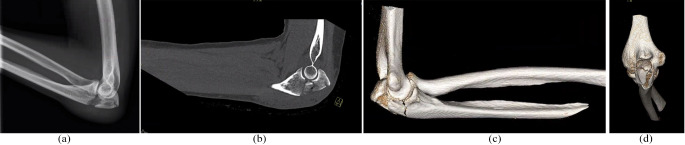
Preoperative imaging of a 47-year-old female with a comminuted and displaced Mayo II-B olecranon fracture (case 24, Table 1). (a, b) Lateral radiograph and sagittal computed tomography (CT-) scan imaging; (c, d) lateral and dorsal view of a three-dimensional reconstruction of the CT-scan
